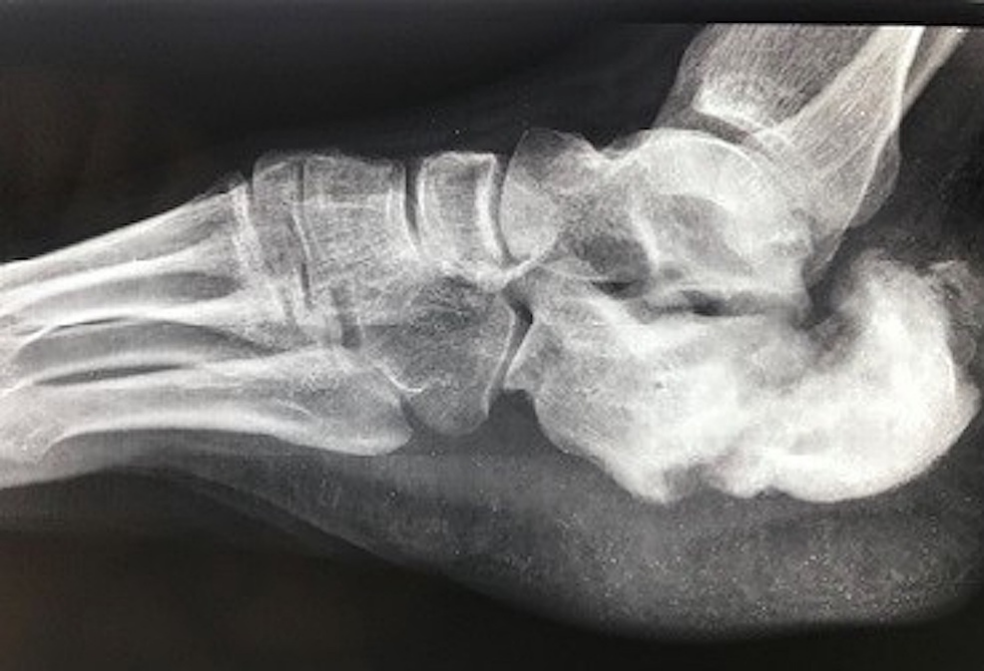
Radiograph of the right foot showing a displaced fracture of the calcaneum with irregular and sclerosed fracture margins.
